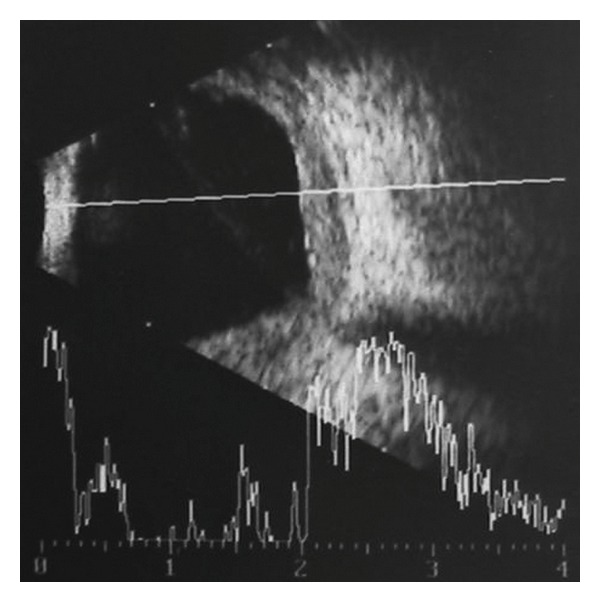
Ultrasound image of the posterior pole of the left eye. Note the thickening of the choroid, compatible with a diffuse choroidal hemangioma.
